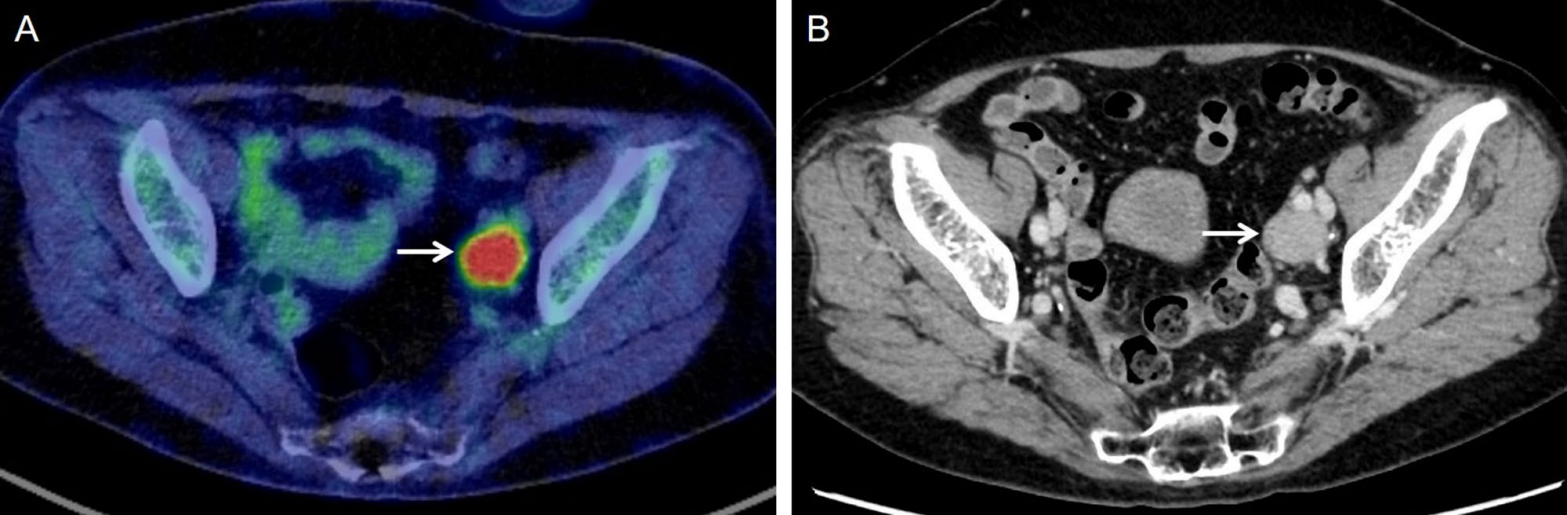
A 72-year-old woman with stage IIIC1 cervical squamous cell cancer. (A) ^18^F-FDG PET/CT image shows positive lymph nodes on ^18^F-FDG PET in a left pelvic lymph node (arrow). (B) CT image shows the lymph node (arrow) of size 2.08 cm (≥ 1 cm).
